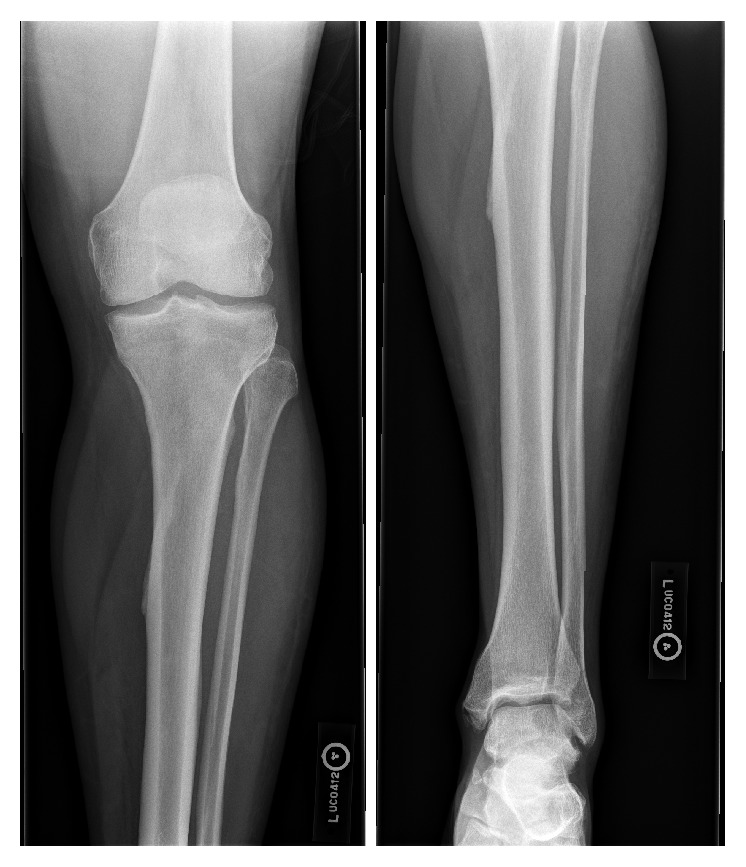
Initial X-ray of the left tibia and fibula is displayed. There is mild tibial plafond spurring. No other acute abnormalities are identified.
